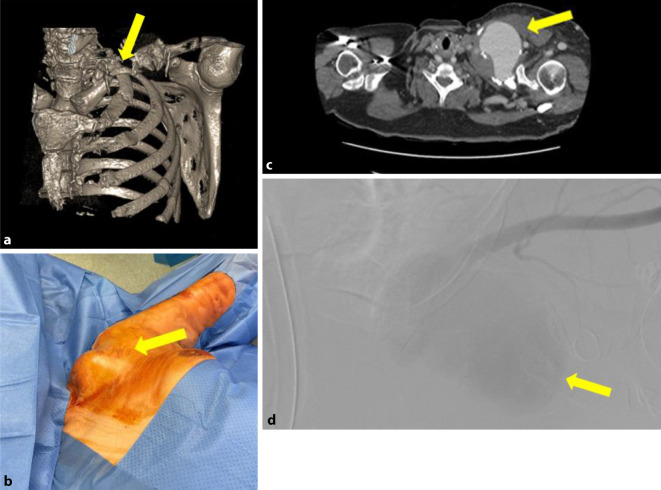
Fall 1: Pseudoaneurysma der A. subclavia links. a 3-D-Rekonstruktion des linksthorakalen CT-Scans, insbesondere der linken Klavikula mit Osteolyse im mittleren Drittel (*gelber Pfeil*), b präoperative Darstellung des linksklavikulären Lokalbefundes mit raumfordernder Wirkung (*gelber Pfeil*), c CT-Angiographie (transversale Schichtung) mit Nachweis des Pseudoaneurysmas links (*gelber Pfeil*), d digitale Subtraktionsangiographie der A. subclavia sinistra mit Nachweis des Pseudoaneurysmas (*mattgrau* – *gelber Pfeil*)
